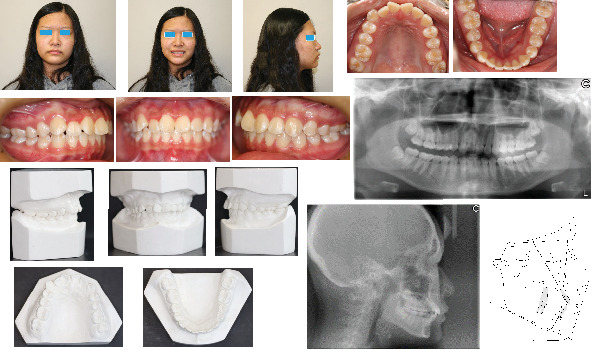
Pretreatment extra- and intraoral photographs, models, radiographs, and cephalometric tracing (black).
